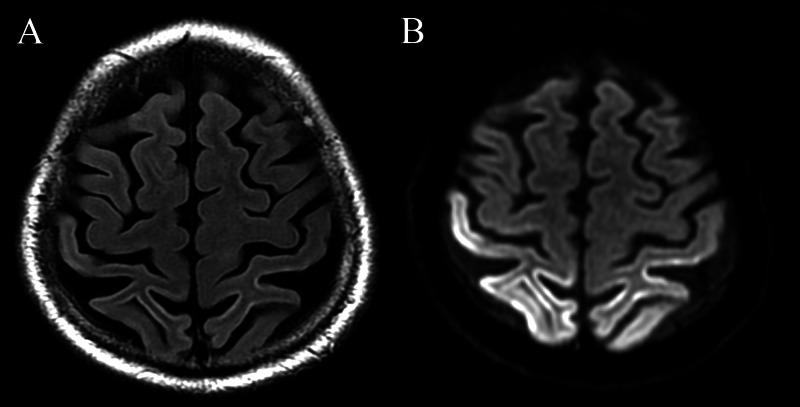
Outside MRI from one year prior to current presentation. (A) Axial FLAIR and (B) diffusion-weighted imaging show areas of diffusion restriction and corresponding FLAIR hyperintensity involving the bilateral peri-Rolandic cortex, greater in the right hemisphere. FLAIR, fluid-attenuated inversion recovery
